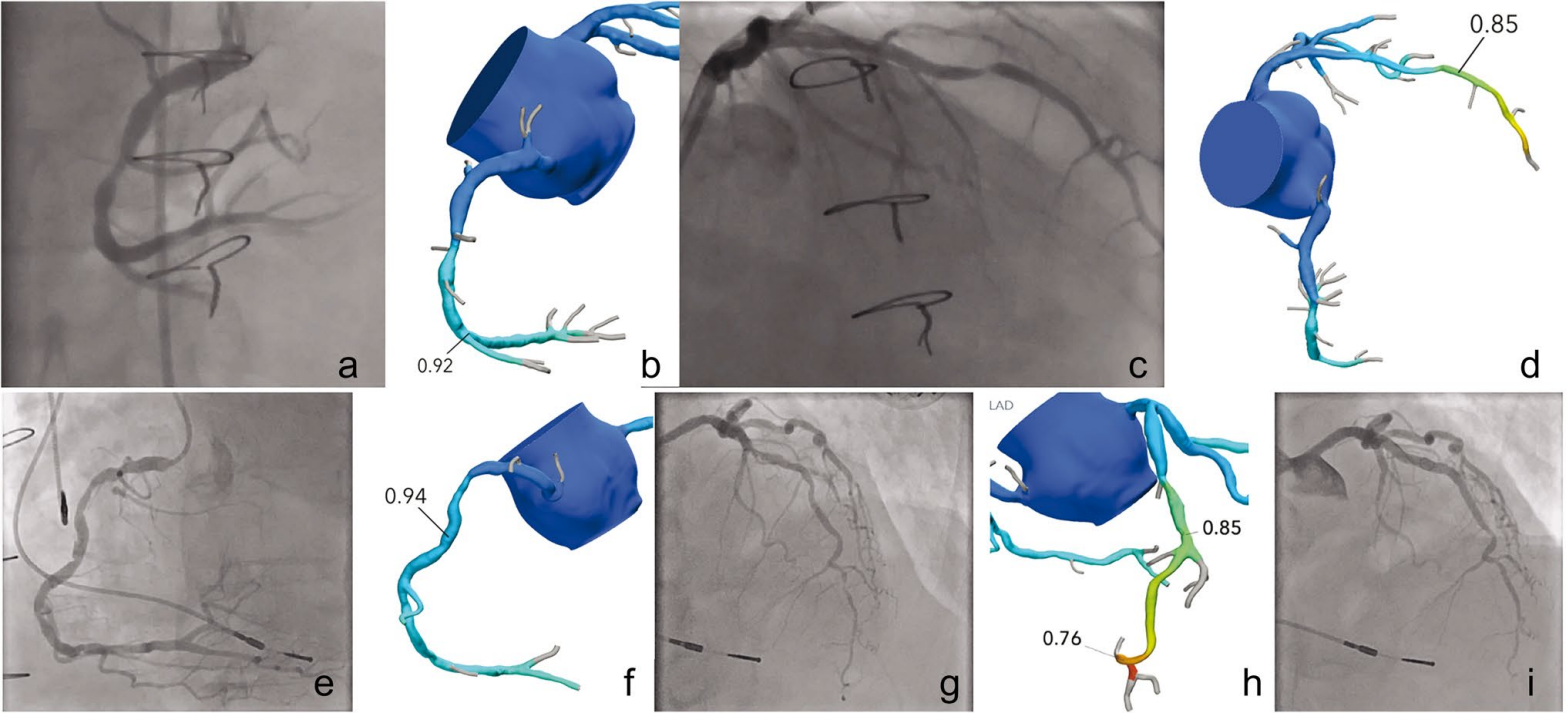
Patient with a focal stenosis in the right coronary artery (RCA) and mid left anterior descending (LAD) of > 30% with FFRct value of 0.92 and 0.85, respectively (b, d). Invasive coronary angiography (ICA) was performed that confirmed the FFRct finding of non-significant stenosis with an invasive FFR value of 0.96 in the RCA and 0.83 in the LAD (a, c). Patient with a focal stenosis in the RCA and LAD of > 30% with FFRct value of 0.94 and 0.76 respectively (f, h). ICA confirmed the lesion in the RCA to be non-significant (invasive FFR 0.95) and stenoses in the LAD (e, g). Additional stress scintigraphy demonstrated ischemia in the LAD territory and the LAD was stented in a subsequent session with good result (i)
